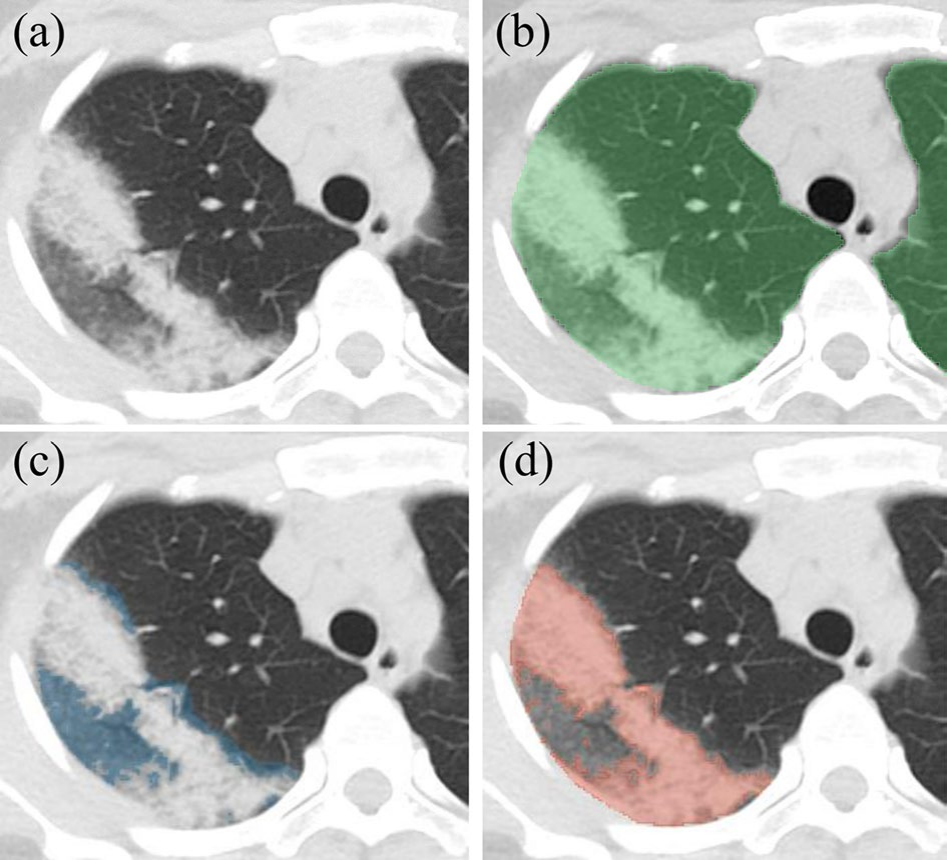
Axial CT images of COVID-19 opacity in right upper lobe, resulting in a part of GGO and a part of consolidation. (a) Non-contrast axial chest CT passing through right upper lobe opacity. (b) Overlaid AI whole lung segmentation (green). (c) Overlaid GGO segmentation alone (blue). (d) Overlaid consolidation segmentation alone (red).
